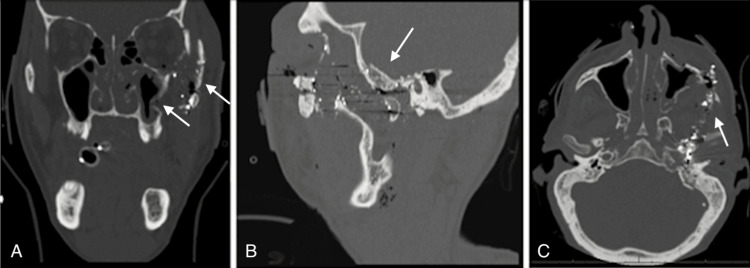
Preoperative CT images of left ZMC fractures (white arrows) in coronal (A), sagittal (B), and axial (C) views CT: computed tomography; ZMC: zygomaticomaxillary
